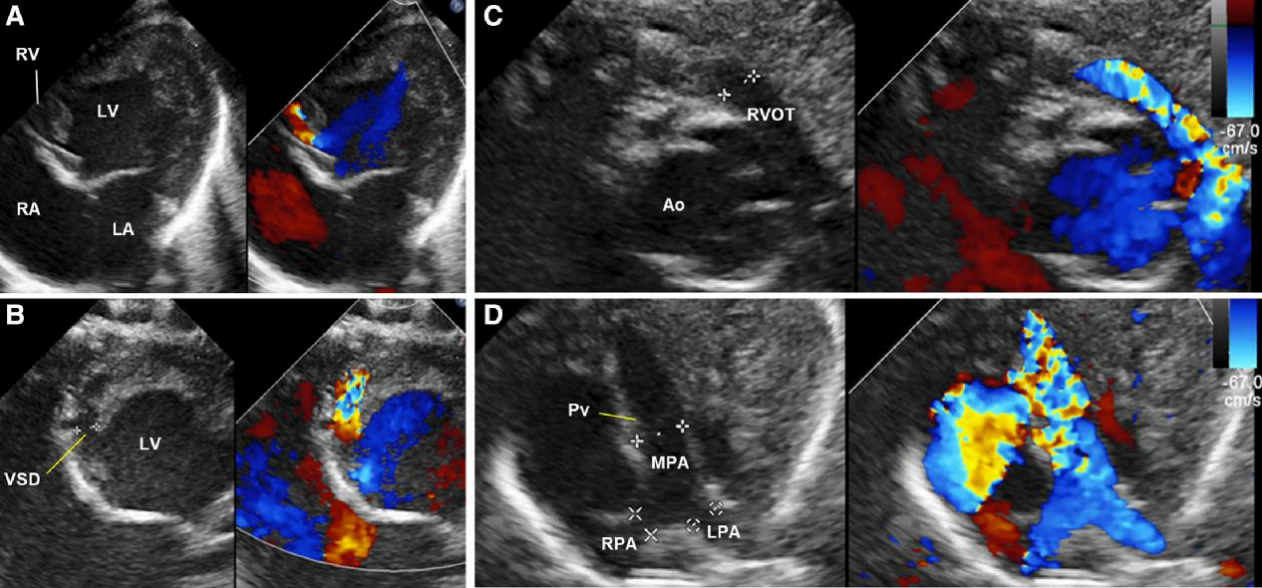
Transthoracic echocardiography after birth. (*A*) Tricuspid atresia and hypoplastic right ventricle, (*B*) a restrictive ventricular septal defect, (*C*) severe right ventricle outflow tract obstruction, and (*D*) hypoplastic main pulmonary artery. No ductus arteriosus could be seen. Ao, aorta; RV, right ventricle; RVOT, right ventricle outflow tract; Pv, pulmonary valve.
